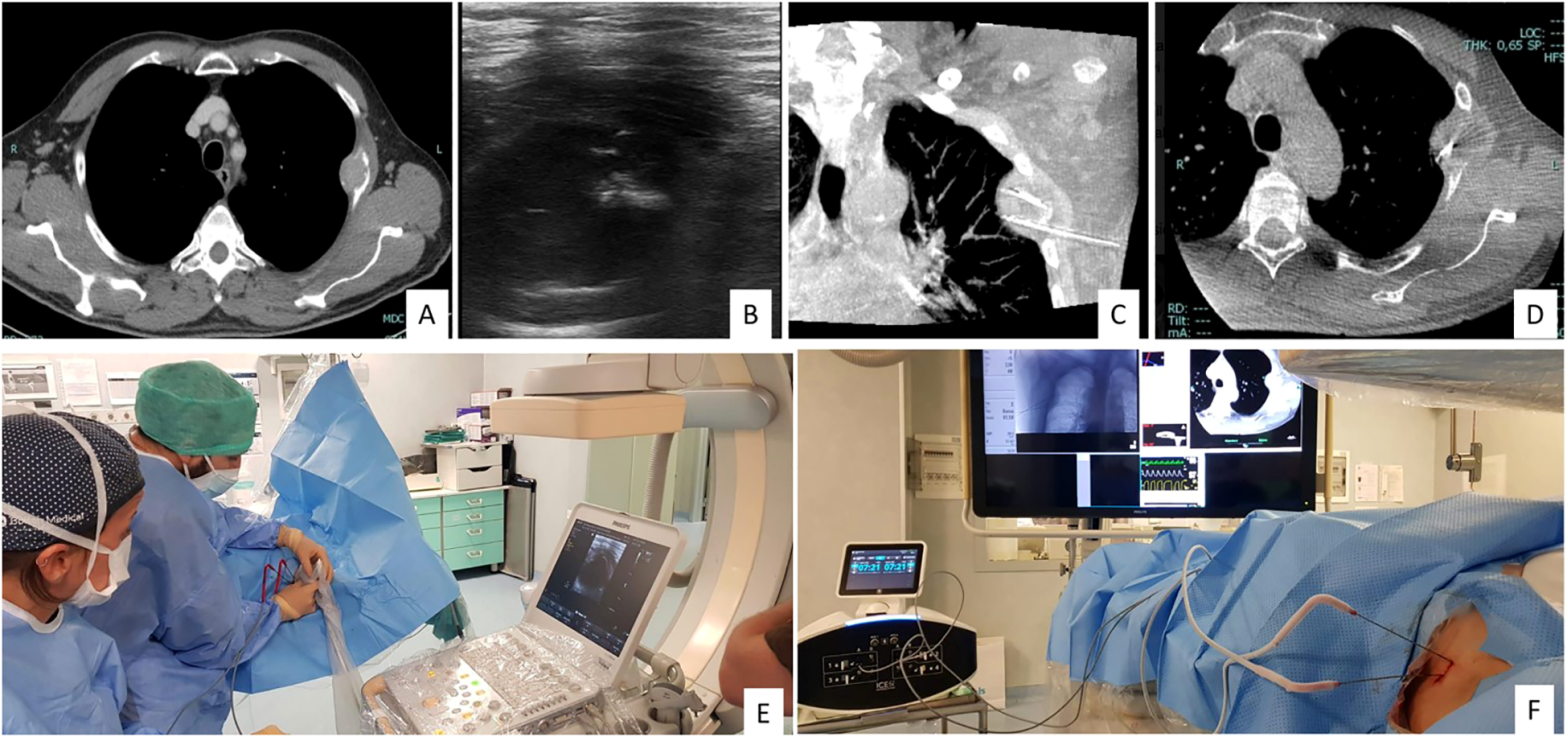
60-year-old man with pleural metastases and osteolytic bone lesion on CT (A). Two antennas for cryoablation (IceCure medical HQ) were placed in the lesion for 4 minutes under US and CBCT guidance (B-F).
